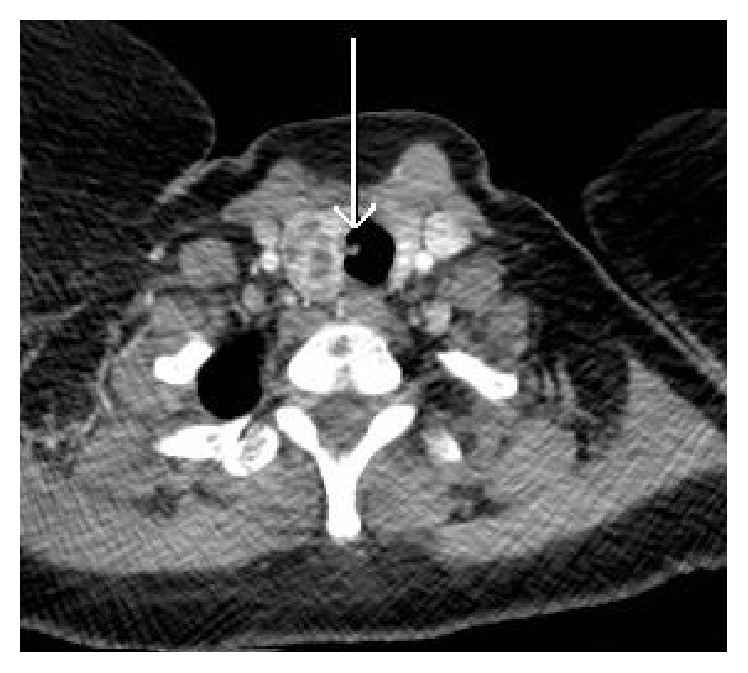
Computed tomography scan demonstrating a pedunculated lesion (arrow) projecting from the right tracheal wall into its lumen.
